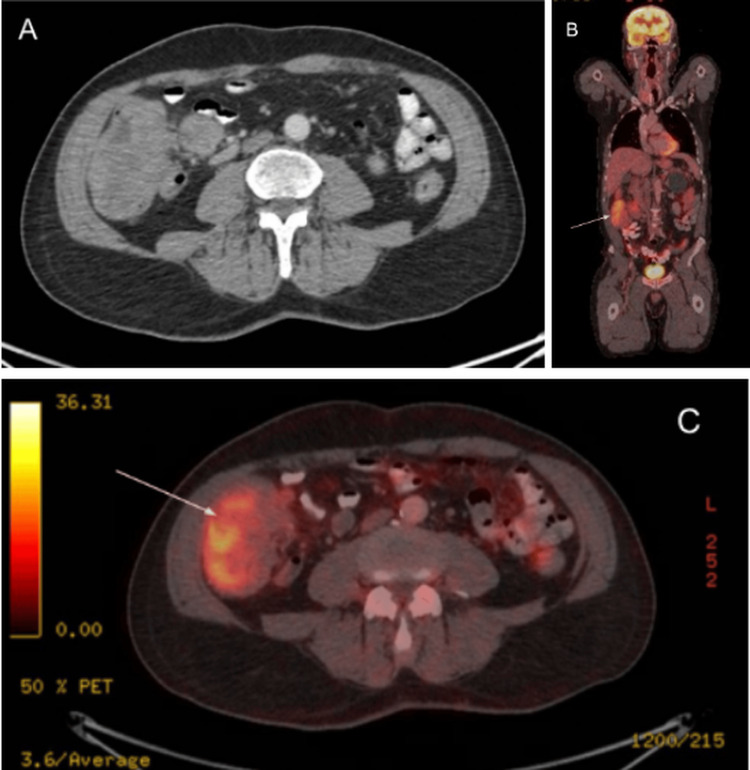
Positron emission tomography-computed tomography scan. (A) Normal small bowel loops. (B and C) Cecal solid heterogeneous lesion measuring 83 mm (maximum standardized uptake value: 7.1) with necrosis and photopenia (white arrows); associated perilesional lymphadenopathies up to 36 × 40 mm (maximum standardized uptake value: 4.7).
